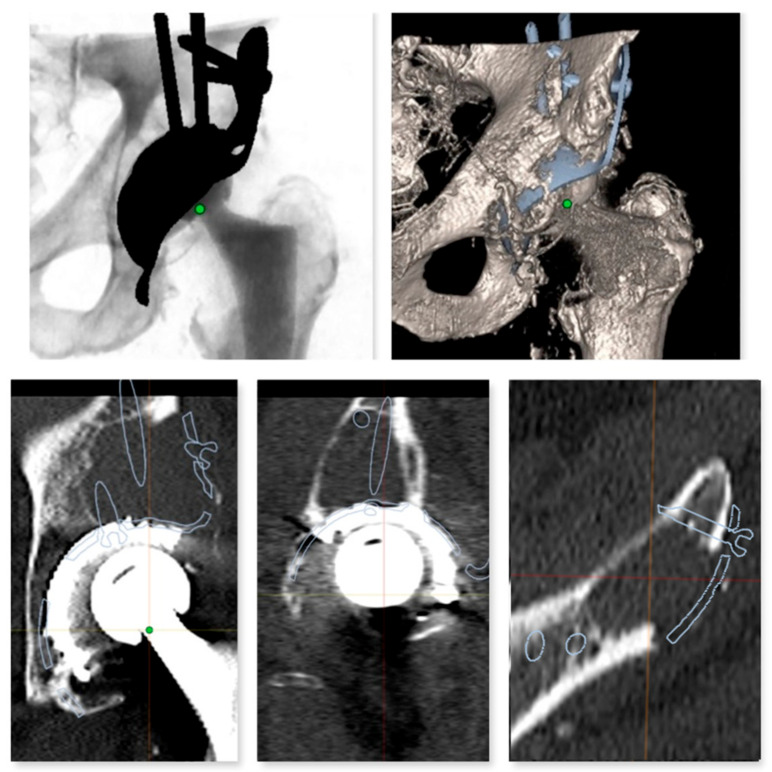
Planning of the screw fixation of the Burch Schneider reinforcement cage. The optimal screw positioning was preoperatively planned by 3D CT imaging.
